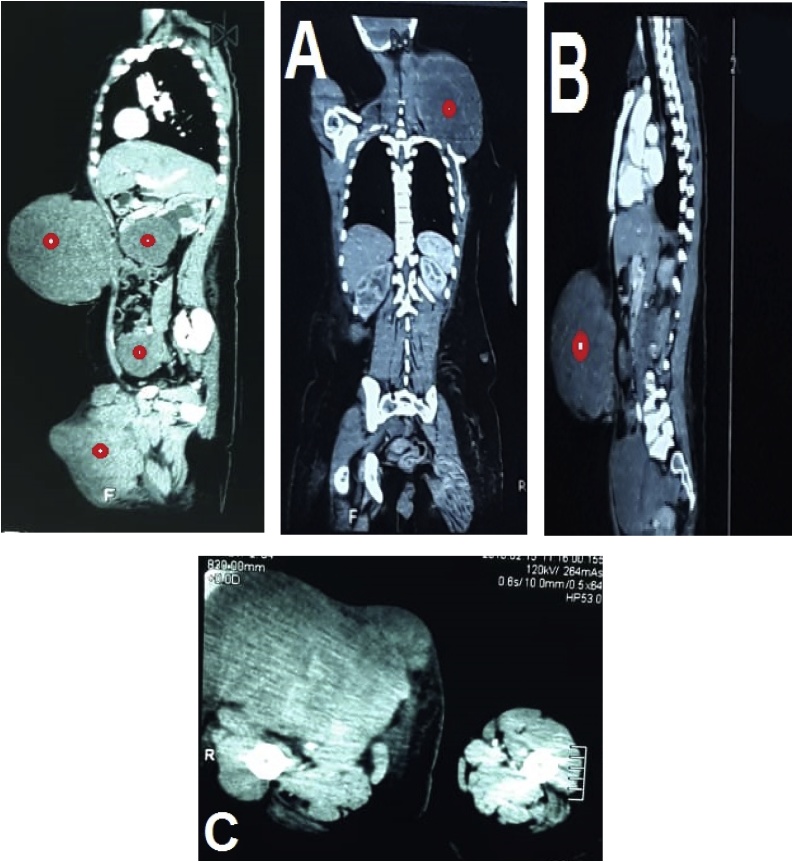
CT scan demonstrating four homogenous masses with low density consisting with liposarcoma. A. The left laterocervical mass measuring 12 × 22 × 18 cm. B. The subcutaneous and retroperitoneal masses that measure 23 × 19 × 16 cm, 8 × 8 × 8 cm respectively. C. The upper outer right thigh measuring 20 × 21 × 25 cm.
